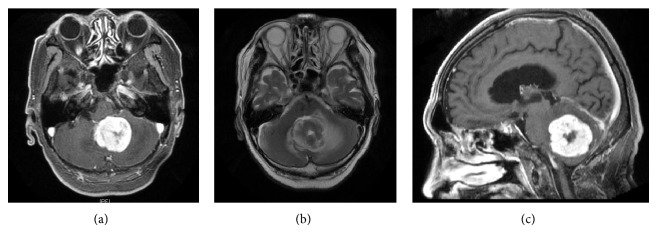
T1-weighted (a, b) and T2-weighted (c) magnetic resonance imaging (MRI) revealing the tumor in the vermis with homogeneous enhancement by gadolinium. Note that the tumor compressing medulla resulting in obstructive hydrocephalus (b).
